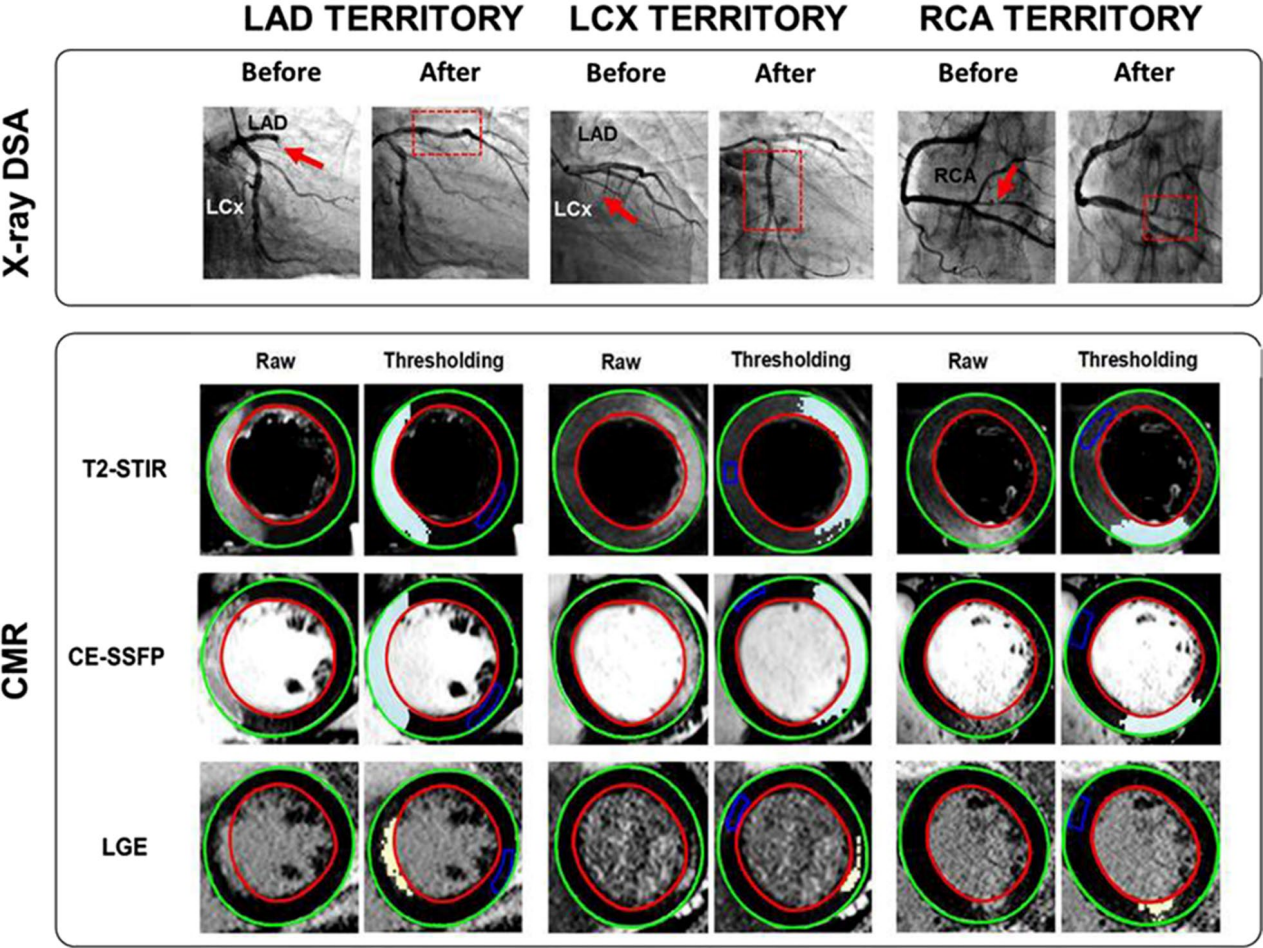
Representative Images from non-ST elevation myocardial infarction (NSTEMI) Patients with left anterior descending (LAD), left circumflex (LCx) and right coronary artery (RCA) myocardial infarctions. X-ray Digital subtraction angiography (DSA) images acquired before and after PCI, along with corresponding short-axis myocardial T2-STIR and contrast enhanced (CE) steady state free precession (SSFP) showing at-risk regions and infarct territories (late gadolinium enhancement (LGE)) acquired at 3T are shown. On X-ray DSA images, the red arrows refer to occlusive or substantially narrowed vessels, and the red box with dotted line demonstrates the re-establishment of blood flow following recanalization of the corresponding vessel. On CMR images, epicardial and endocardial borders are delineated by green and red borders, respectively. The left columns shows the raw images and the right columns shows the same images processed using semi-automatic threshold-based signal detection. The edematous myocardium delineated by the T2-STIR and CE-SSFP images are shown as a region enclosed by the pale blue areas and irreversibly damaged myocardium is identified on LGE images as yellow areas
